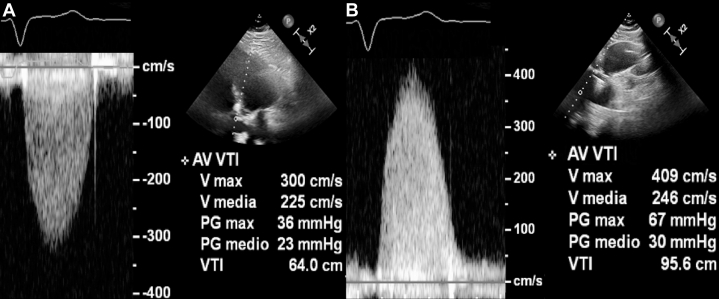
Aortic Bioprosthesis Degeneration The right parasternal approach (B) revealed higher gradients through the bioprosthesis vs the apical view (A).
